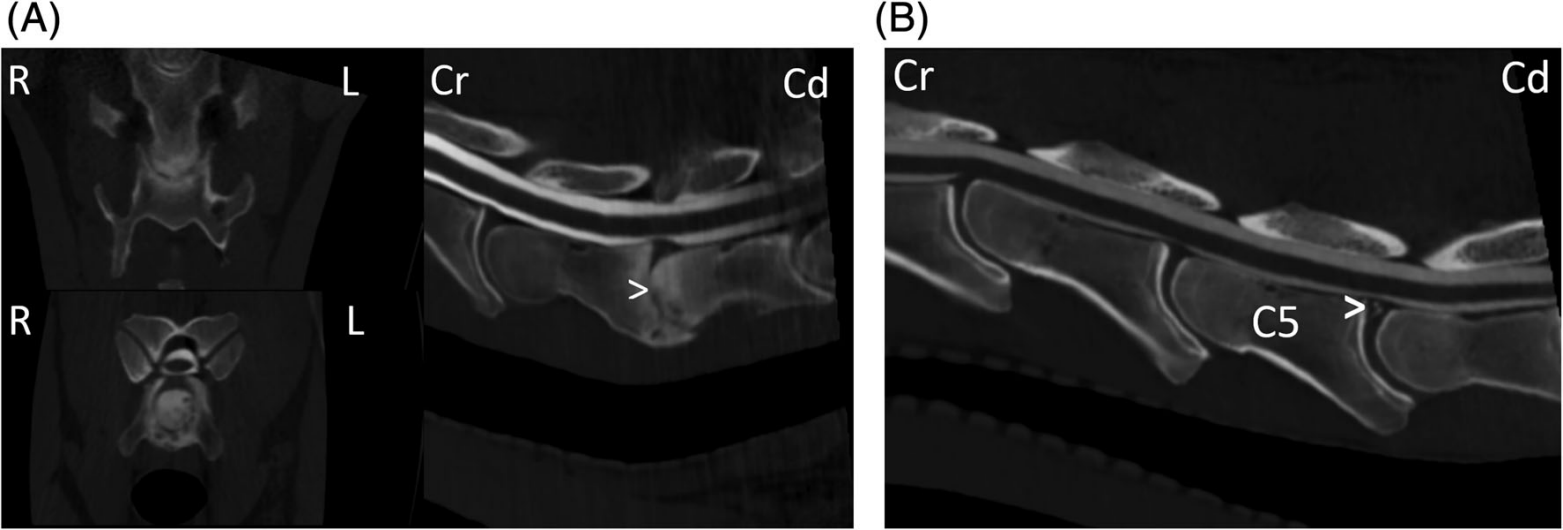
A, Computed tomographic (CT) myelographic 3D multiplanar reconstruction at the level of C6‐C7 in case 16. Note the collapse of the intervertebral disc space (white arrowhead) with severe surrounding increased bone attenuation, and multiple rounded hypoattenuating defects within the vertebral end plates. B, Saggital plane CT myelographic reconstruction at the level of C5‐C6 in case 8. The white arrowhead indicates mineralization within the dorsal portion of the intervertebral disc, not causing spinal cord compression
